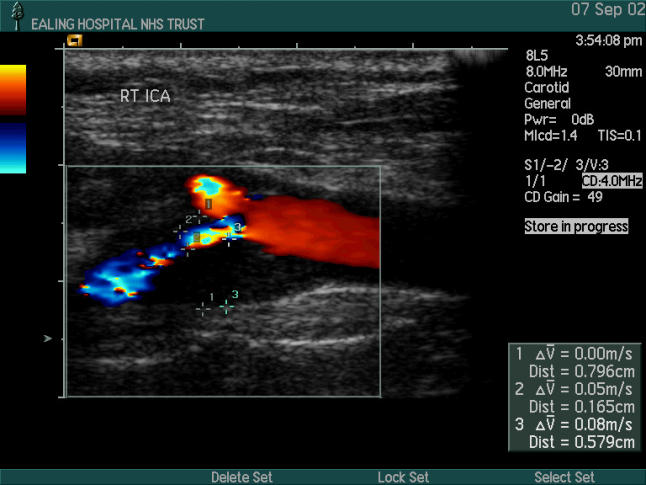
Carotid Artery Ultrasound Showing an Echolucent Atherosclerotic Plaque (Geroulakos Type 2) This plaque is causing approximately 80% stenosis, as determined using diameter reduction and velocity ratio methods.
